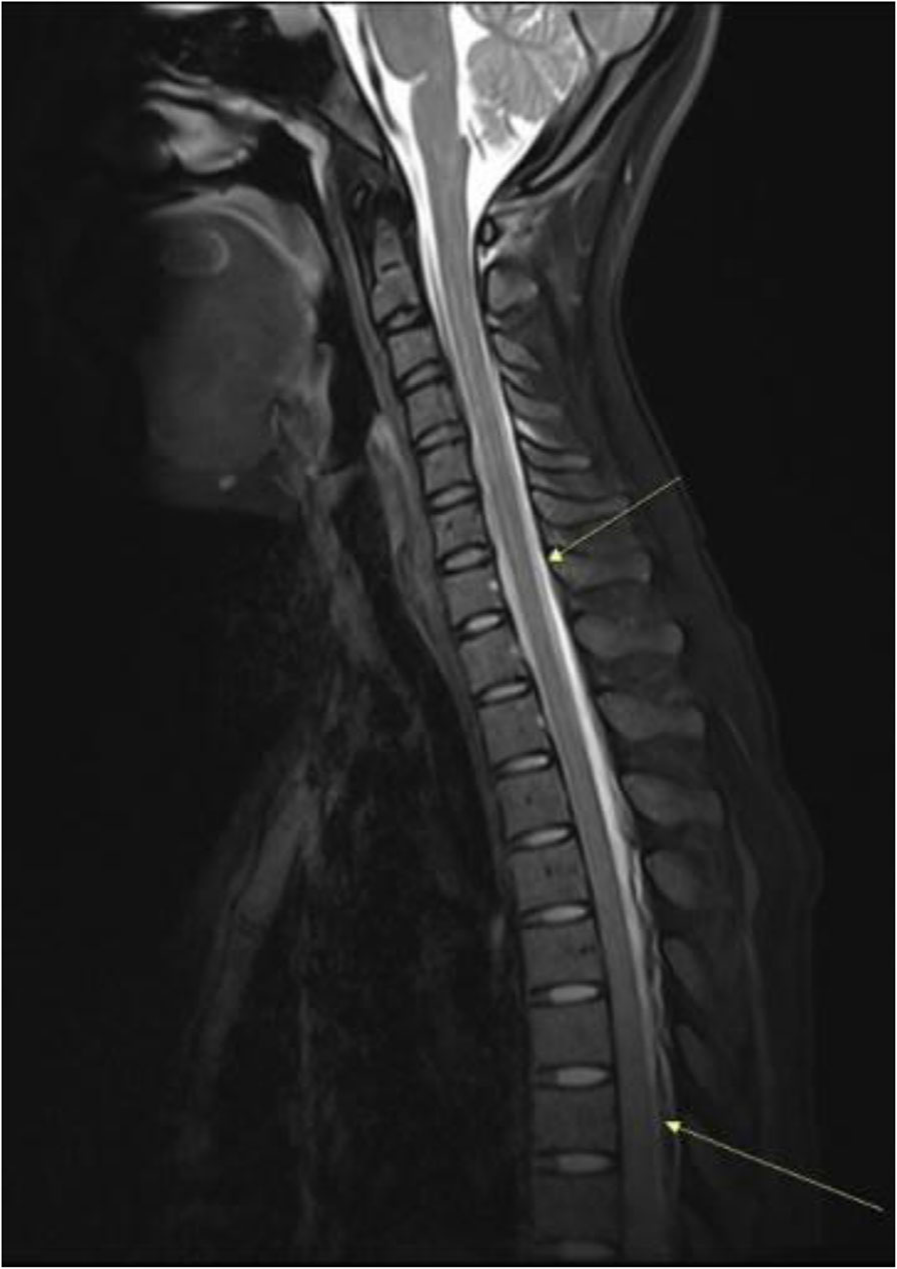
Spine MRI show diffuse fluid collection in the epi and subdural space, posterior to the C7-T7 cord. (arrows)
